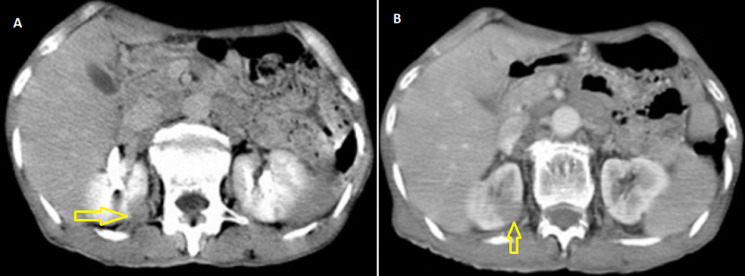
A) abdominal CT scan showing a right renal mass (arrow); B) low significant enhancement (arrow)
